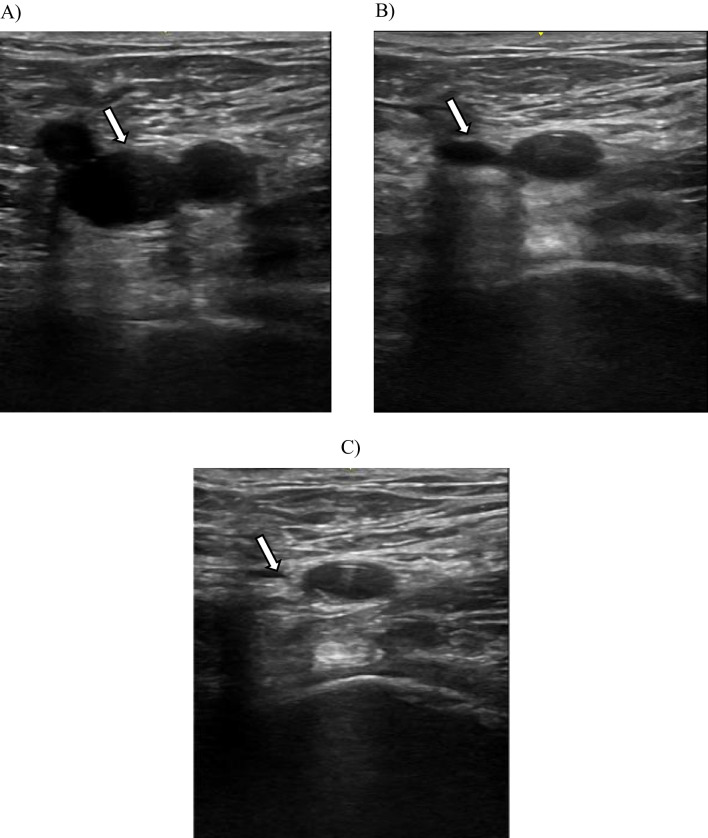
Ultrasound Image by IPCUS. A) Normal appearance of left common femoral vein; B) Partially compressed left common femoral vein; C) Completely compressed left femoral vein
